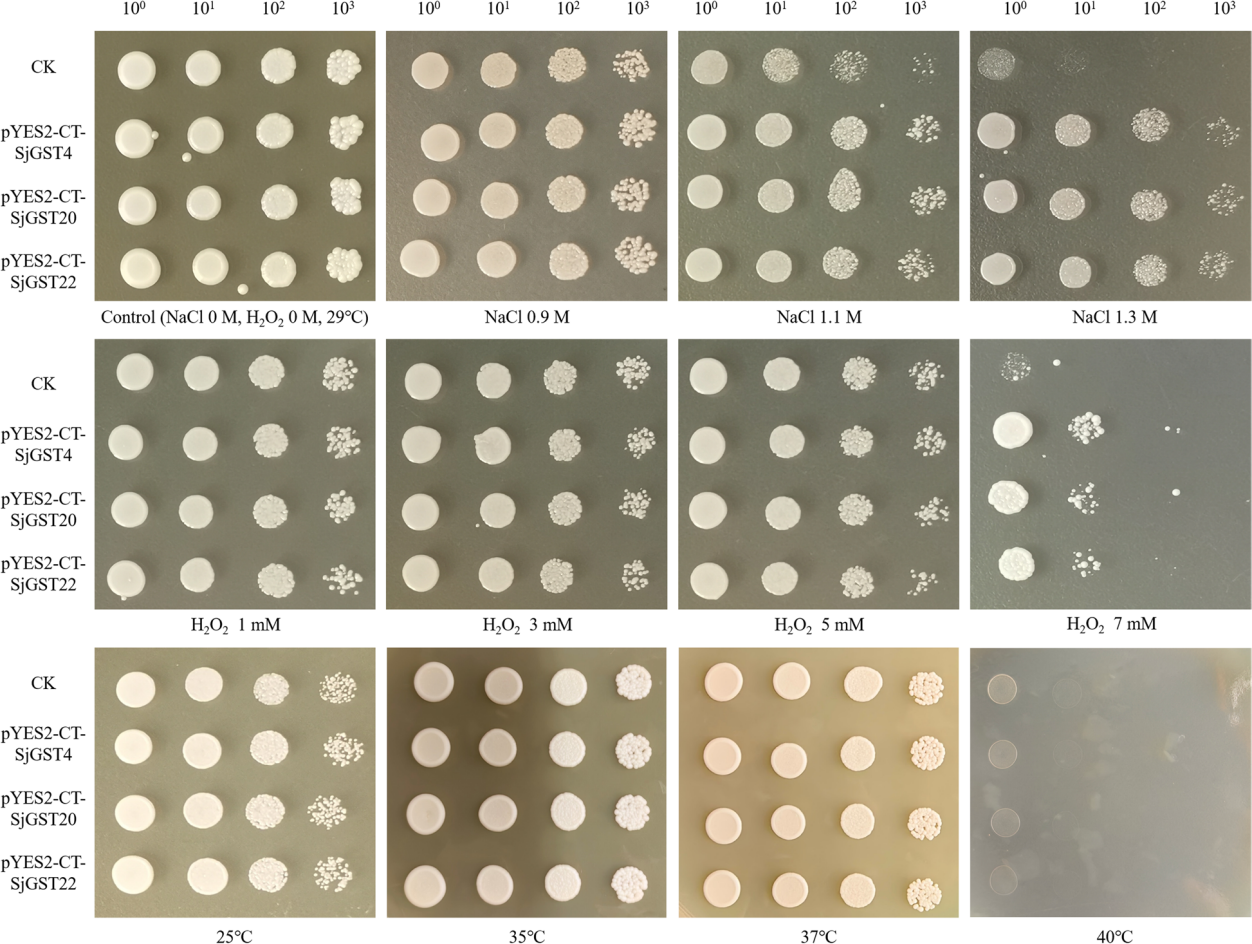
The growth pattern of CK and recombinant strain pYES2-CT-SjGST4, pYES2-CT-SjGST20 and pYES2-CT-SjGST22 on YPD plates for 4 d at different concentration NaCl, H_2_O_2_ and temperatures. CK: INVSc1 transformed with an empty pYES2-CT vector. Control: YPD plate with 0 M NaCl and 0 M H_2_O_2_ cultured under 29℃. 10^0^–10^3^: dilution ratio of yeast culture solution
